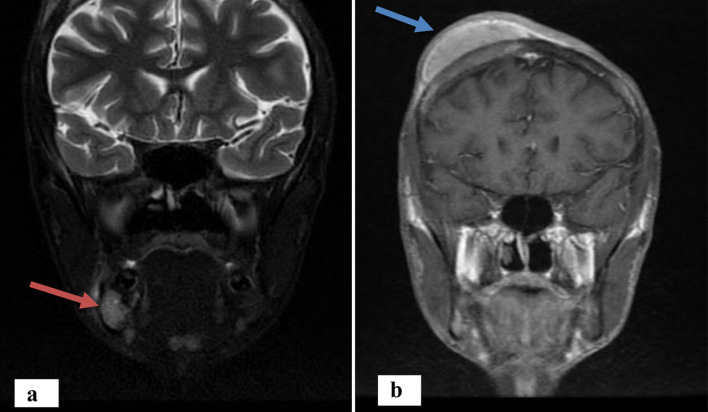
Brain and face MRI of the patient. (a) Osteomyelitis of the right mandible (red arrow). (b) Diffuse scalp swelling, frontoparietal regions, consistent with soft head syndrome (blue arrow). MRI: magnetic resonance imaging.
